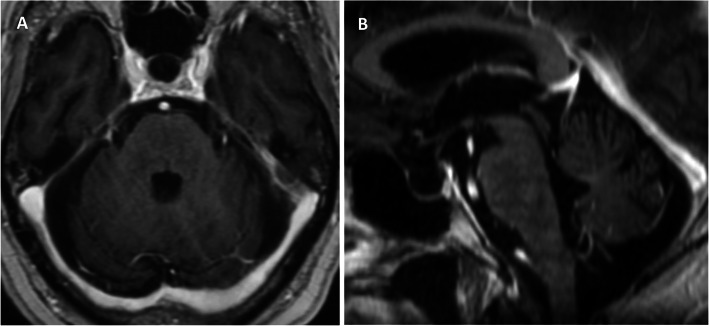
Follow-up MRI brain 4 months post commencing treatment. a Axial and b sagittal T1-weighted post-contrast images showing normal appearance of the pons, cerebellar peduncles and pontomedullary junction with no residual enhancement
